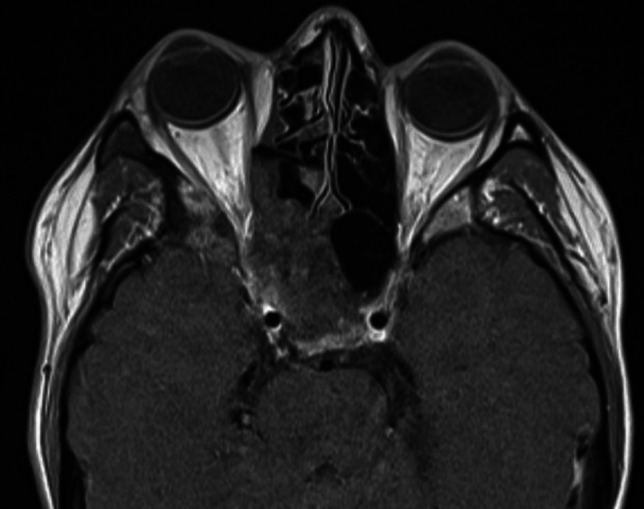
An axial head MRI slice of a 28-year-old female patient with monostotic FD. The image shows a large lesion involving the right frontal bone, ethmoidal bone, maxilla, and the zygomatic bone with subsequent narrowing of the orbit and protrusion of the eye. The patient’s symptoms were facial asymmetry, numbness, feeling of pressure, exophthalmos and ptosis of the right eye and intermittent diplopia
